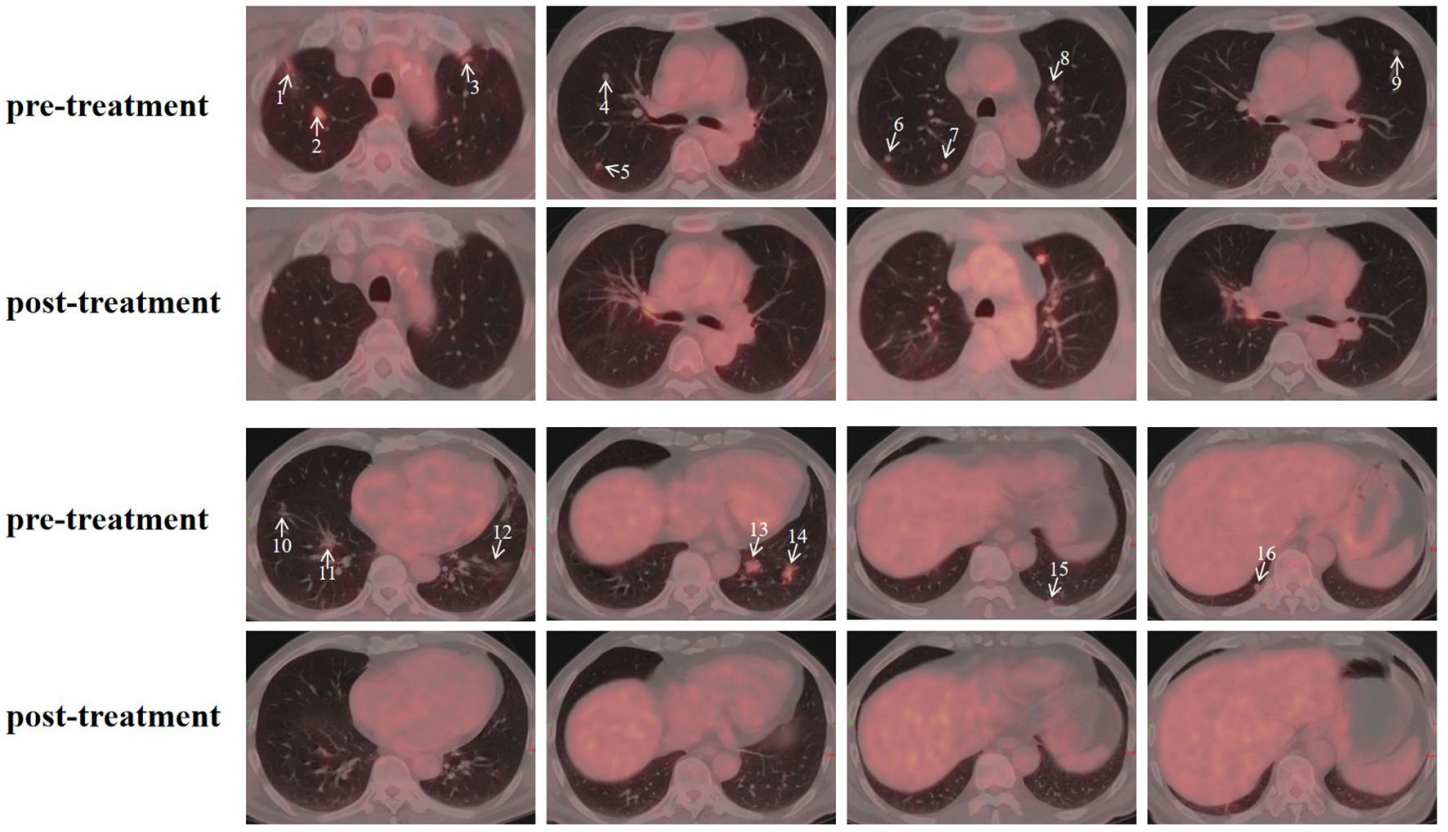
Pre- and post-treatment PET/CT images of pulmonary metastases. Arrows indicate the locations of the metastatic lesions, with numbers corresponding to the lesion IDs in Supplementary Table.
